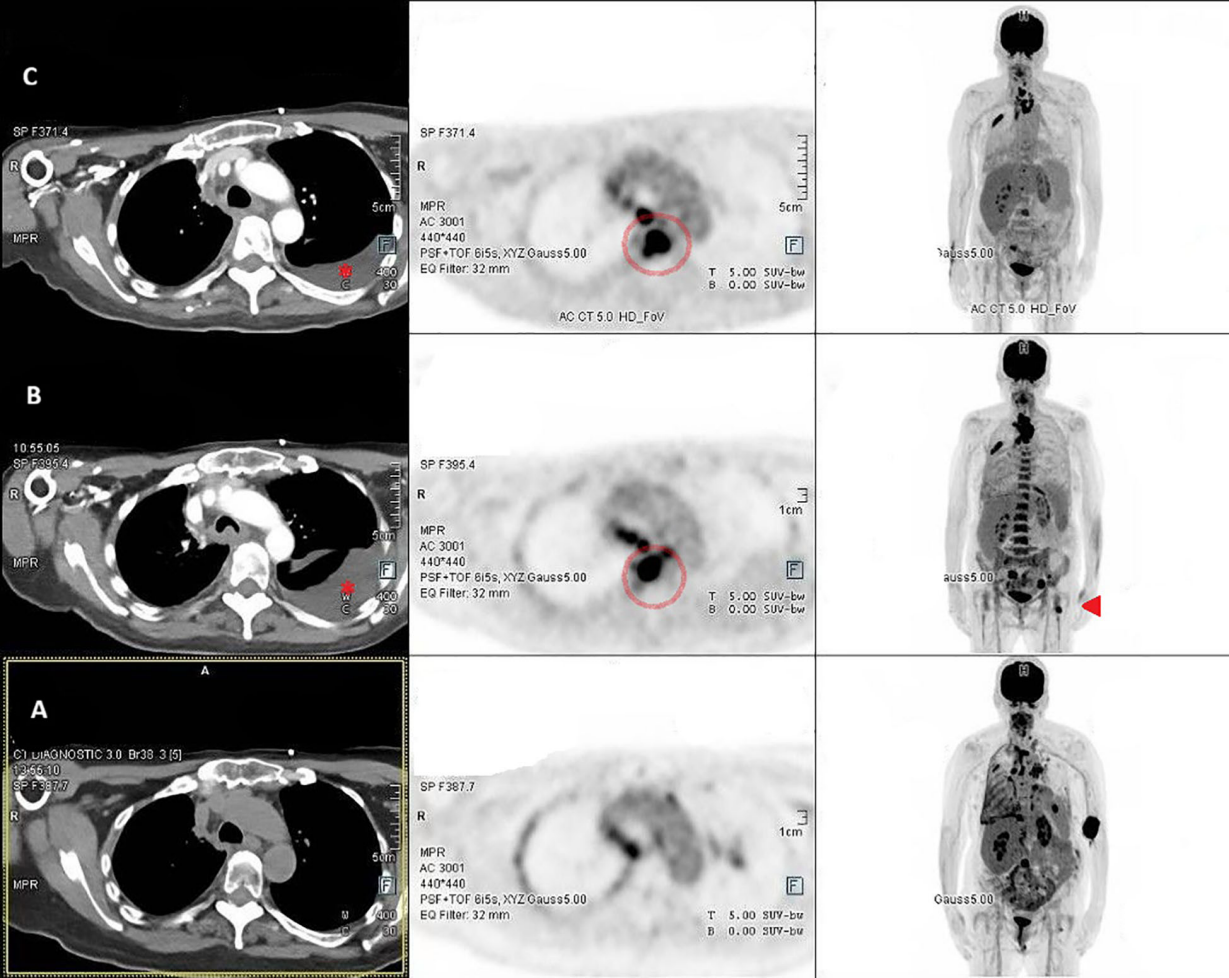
Serial PET-CT images demonstrate the patient’s radiological response and subsequent disease progression. Initial scans [(A), October 2023] show radiological improvement including in the left femur metastasis (arrowhead), following 1 year of therapy on a reduced-dose regimen, coinciding with development and gradual resolution of pleural effusion (asterisk). Later images [(B), April 2024; (C), July 2024] depict disease progression, including the emergence of a D5 vertebral metastasis with associated spinal canal narrowing (circle) and a cervical soft-tissue mass compressing the internal jugular vein. These findings prompted the initiation of cabozantinib therapy. Despite treatment, further progression was observed on follow-up imaging.
